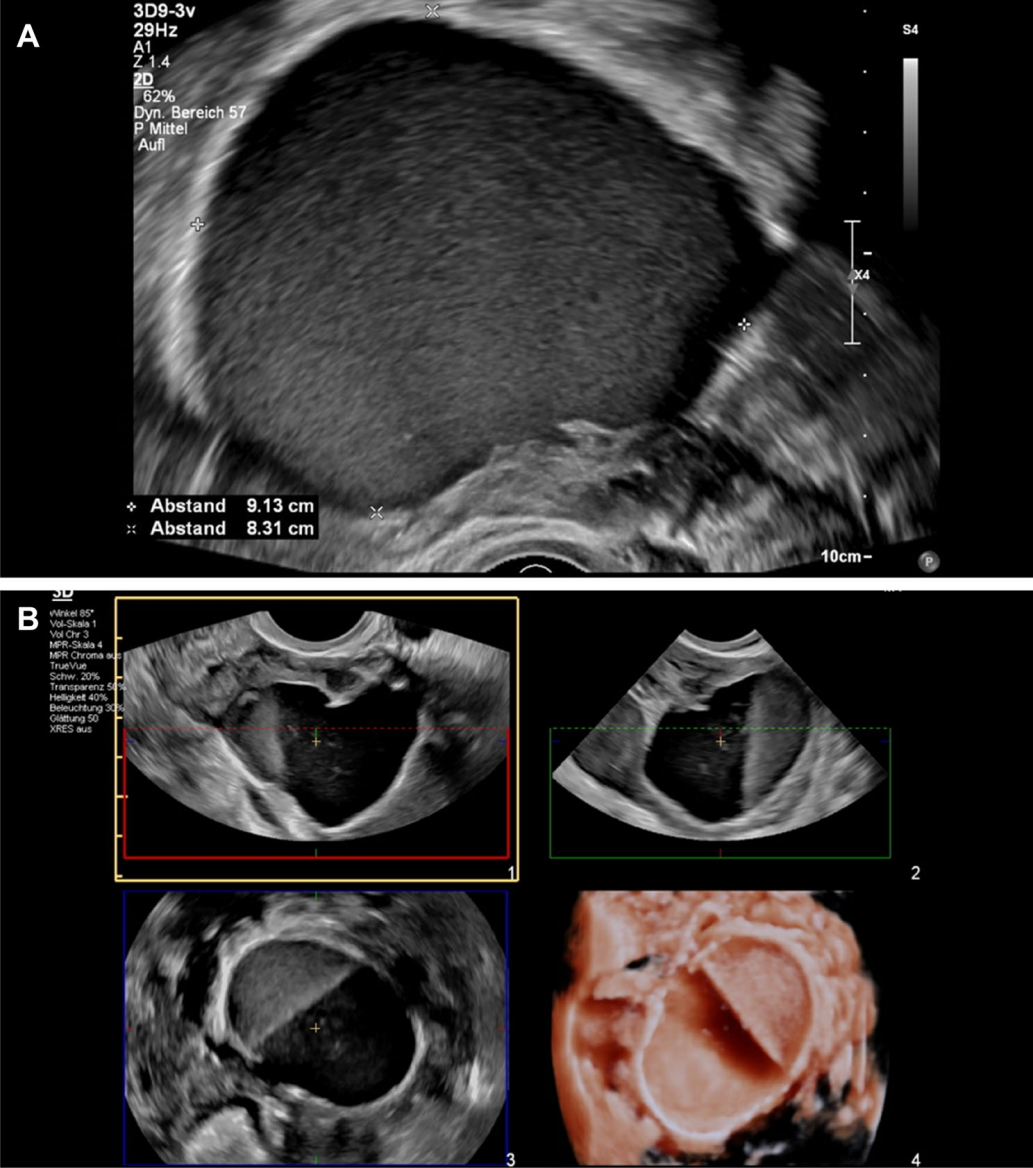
a Typical unilocular endometrioma (diameter > 7 cm = #Enzian(u)O3). Echogenicity: ground glass-like echogenicity. b Sonographic image of an atypical multilocular ovarian endometrioma with different echogenicity of the locules
